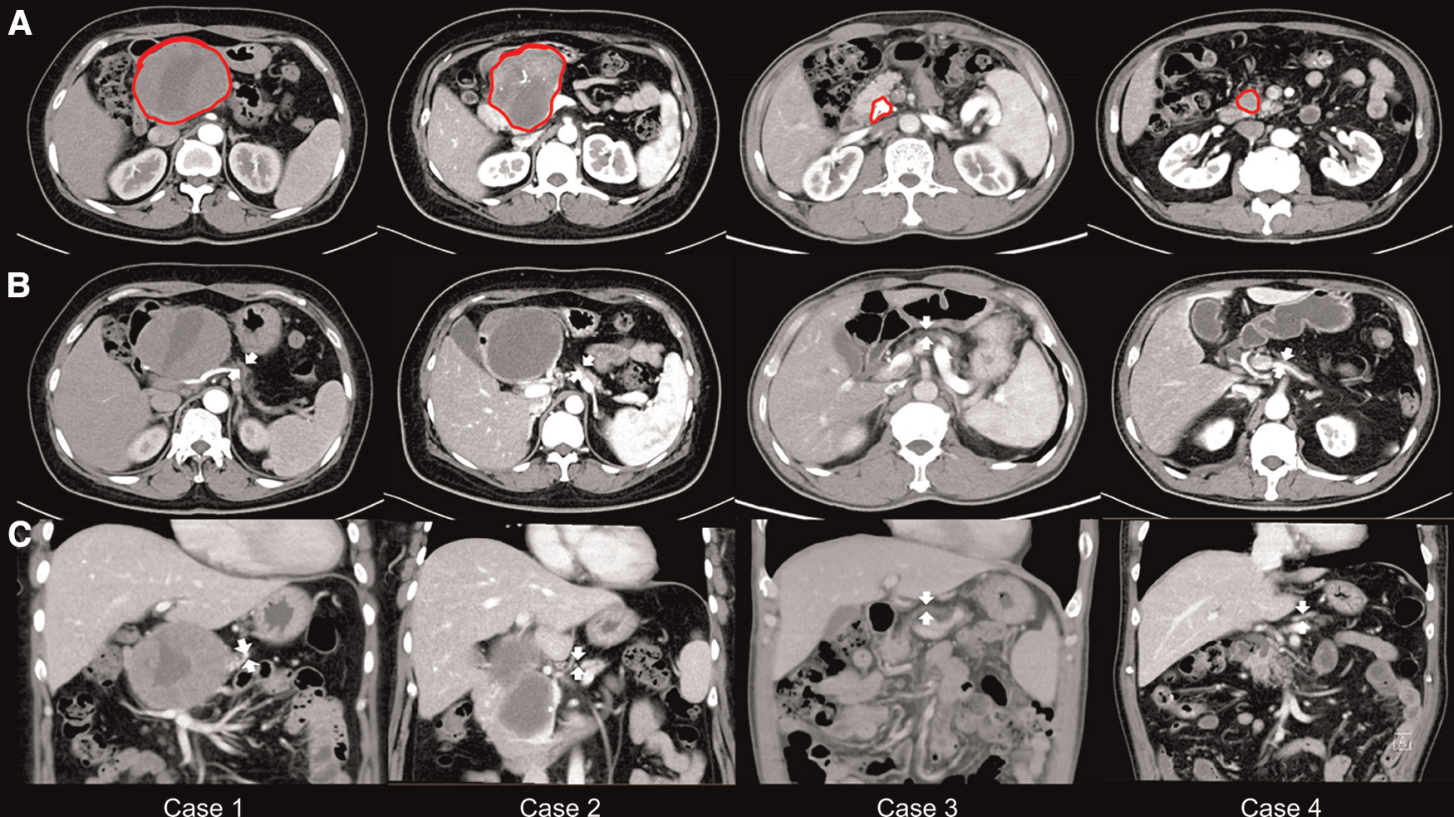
Preoperative abdominal computed tomography scans. (A) The tumor or stone is marked in red. (B) The width of the pancreatic bodies is < 10 mm, with arrows highlighting the atrophy of the pancreatic bodies. (C) The thickness of the pancreatic bodies is < 10 mm in the image, with arrows indicating the atrophy of the pancreatic bodies.
